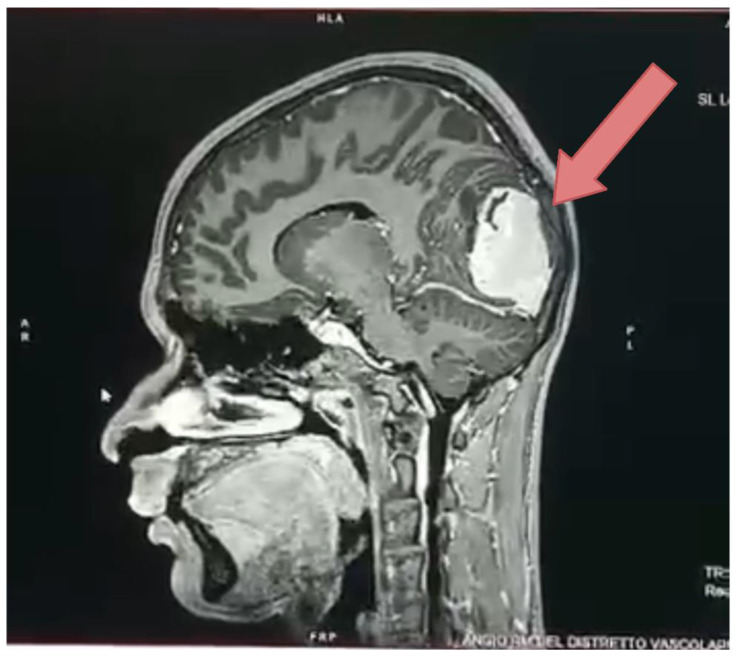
MRI of the brain with contrast enhancement (MDC) and MR angiography of the intracranial arterial circulation revealed a Chondrosarcoma appearing as an isointense mass in the occipital–parasagittal region (as indicated by red arrow), measuring 52 × 53 × 43 mm. The lesion is durally based on the lateral aspect of the confluence of sinuses on the left side, with its inferior margin delineated by the tentorium cerebelli. At its site of attachment, the mass is in close adherence to the left transverse sinus, near the confluence of sinuses, causing approximately 8 mm of compression.
